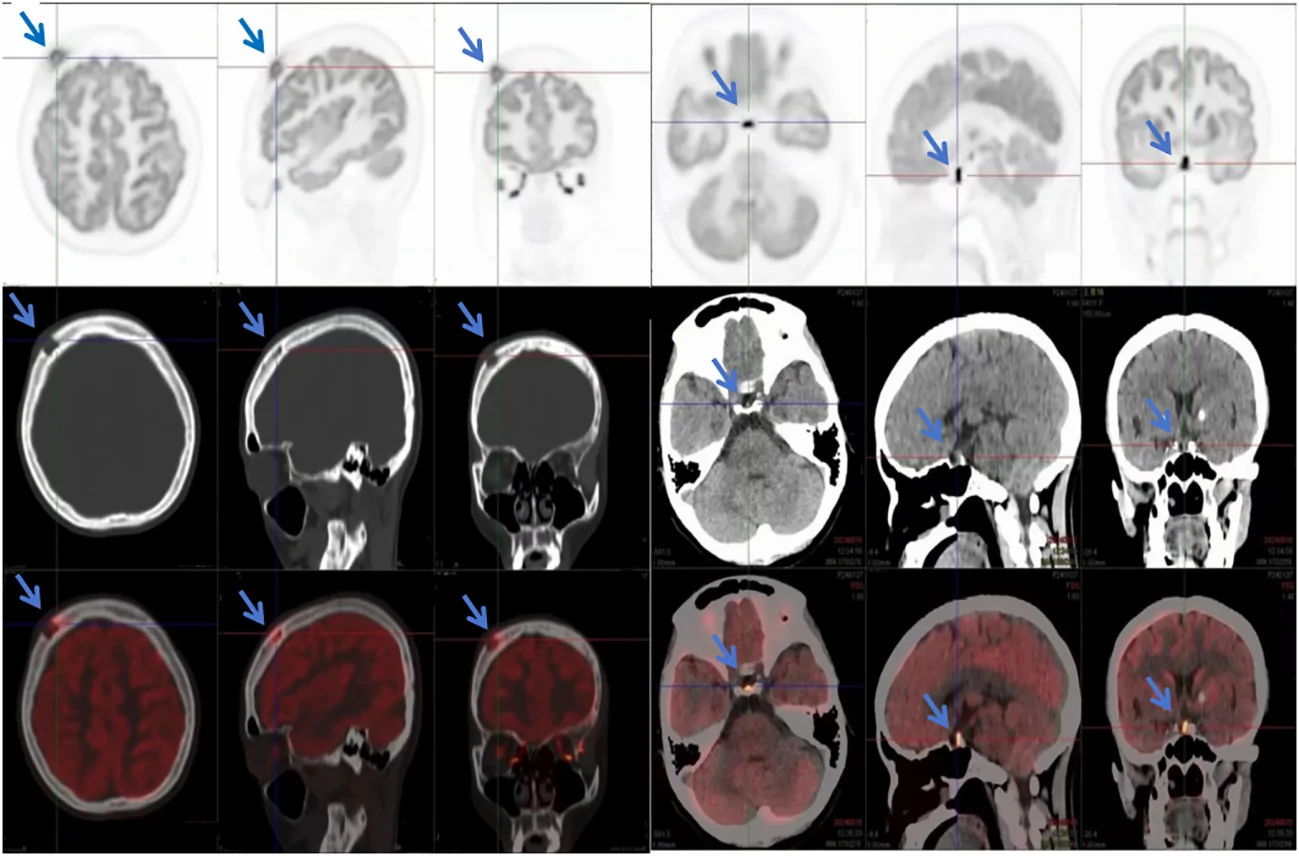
Gray scale = CT brain; Red overlay = FDG-PET hypermetabolism. The areas marked by blue arrows represent hypermetabolic lesions. The left side shows the skull lesion, and the right side displays the pituitary lesion.
